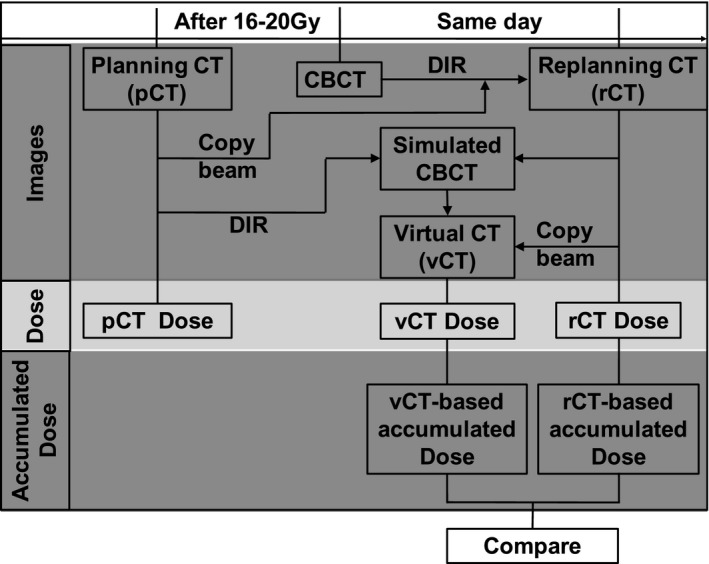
The approach of “dose of the day” calculation based on CBCT and DIR. CBCT, cone beam CT; DIR, deformable image registration.
